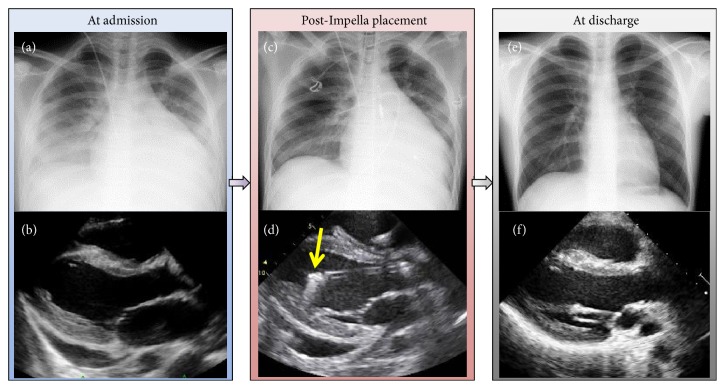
Findings on admission and after Impella placement*. (a) Pre-Impella placement chest radiograph shows prominent pulmonary edema with an enlarged cardiac shadow. (b) At admission (pre-Impella placement), echocardiography, parasternal long-axis view, shows an extensively thickened left ventricle with enhanced echogenicity and a modest amount of pericardial effusion [left-ventricular ejection fraction (LVEF) 42%]. (c) Post-Impella placement chest radiography shows less pulmonary edema. (d) Post-Impella placement echocardiography, parasternal long-axis view, shows Impella inlet parts with an acoustic shadow in the proper position (yellow arrow) (LVEF 27%). (e) At discharge, chest radiography shows an almost normal cardiac shadow. (f) At discharge, echocardiography, parasternal long-axis view, shows improved contractility without pericardial effusion (LVEF 60%).
